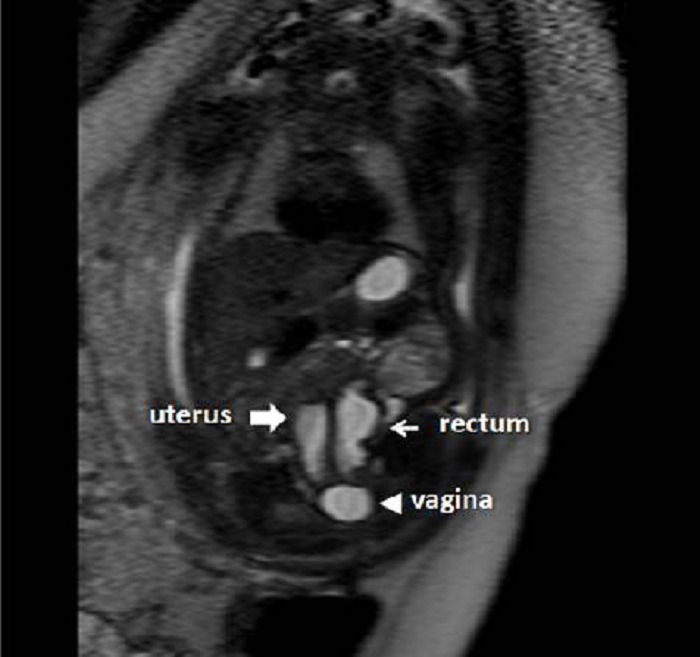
Figure 2A: A dilated vagina, uterus and rectum were demonstrated by fetal MRI. A separate bladder was invisible.
